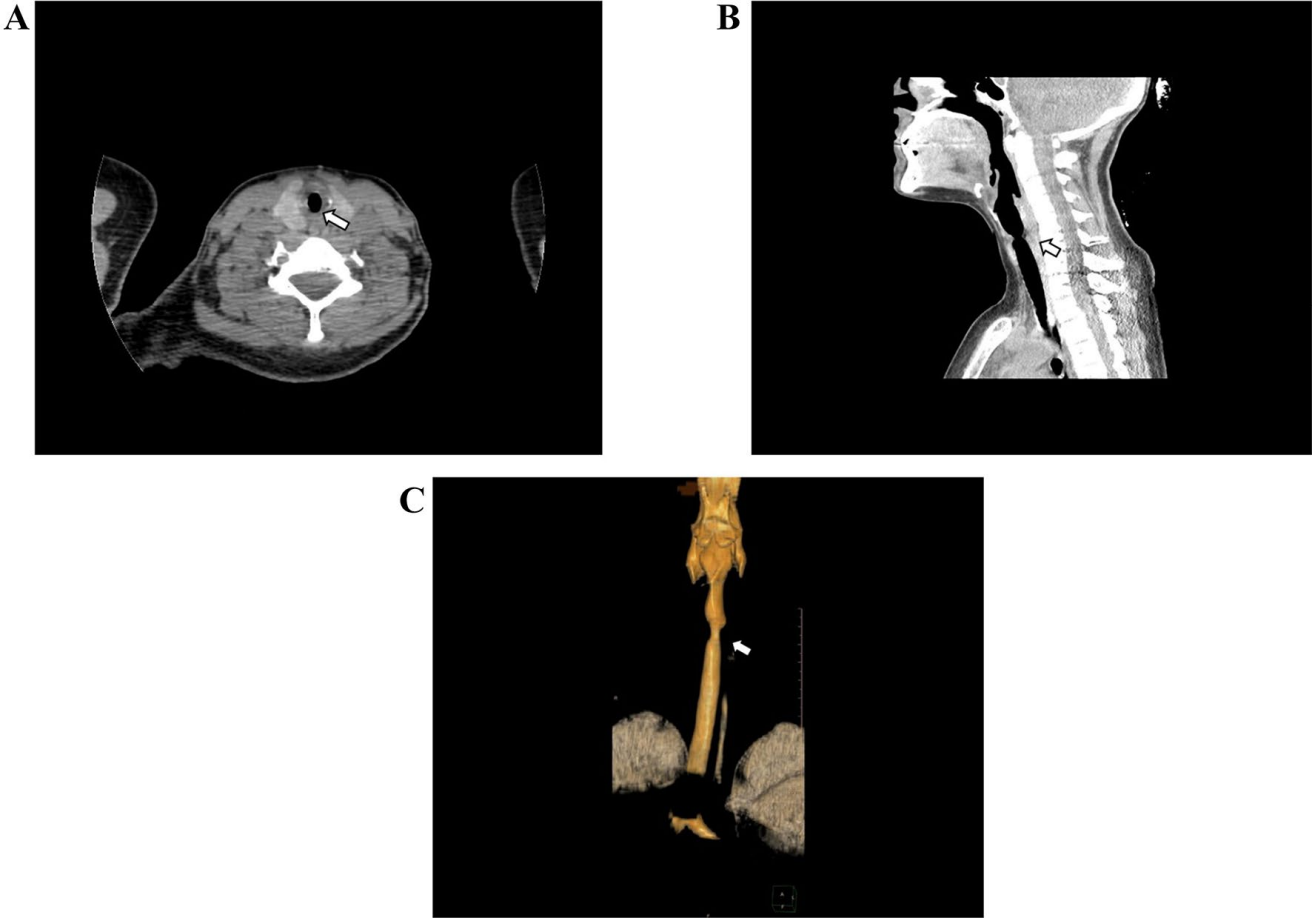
Cervical computed tomography images showing a thickening at the level of the first tracheal ring (arrows), with no apparent origin. a Axial plane. b Sagittal plane. c 3D reconstruction
